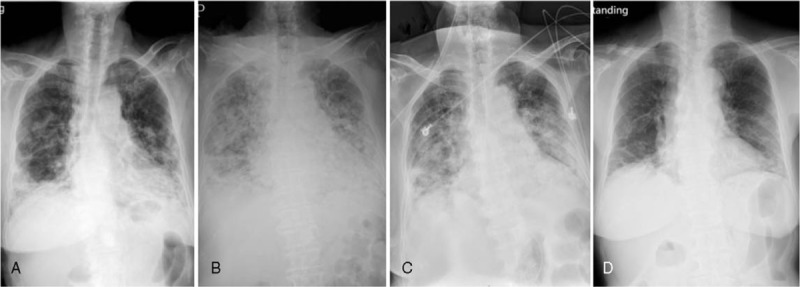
Serial chest X-rays showed: (A) infiltrates and consolidations in bilateral lungs with lower lobe predominance on the first day in the emergency department; (B) deterioration of bilateral lungs, for which noninvasive ventilator support was used; (C) improvement in bilateral infiltrates 1 week after discontinuing mesalazine and the initiation of steroid therapy; (D) resolution of most infiltrates in bilateral lungs about 2 months after the onset of symptoms.
